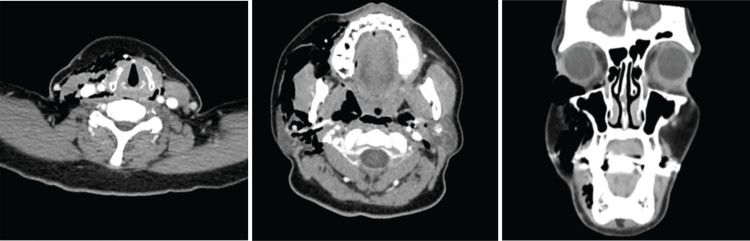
Multiple CT views showing extensive subcutaneous emphysema CT: computed tomography
